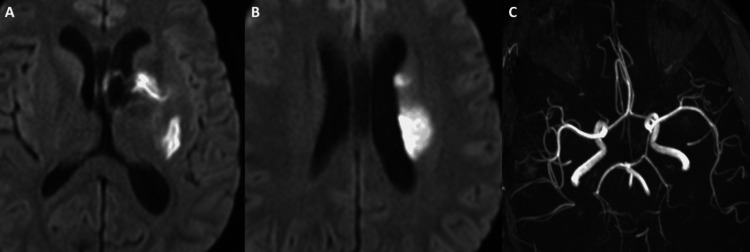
(A, B) Diffusion-weighted imaging (DWI) revealed acute infarct in the left basal ganglia and corona radiata. (C) 3D reconstruction of time of flight (TOF) MR brain angiogram showed no large vessel occlusion or severe stenosis.
